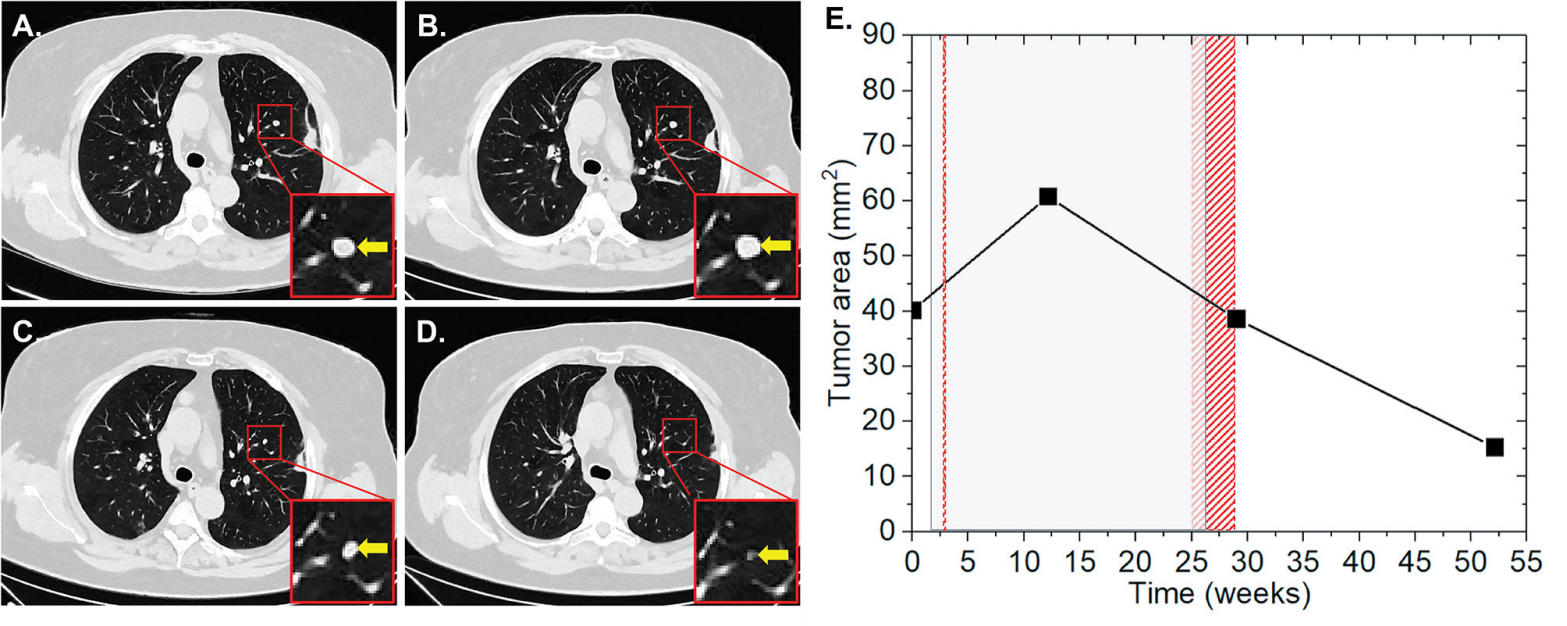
Patient 1 (Table 2B) presenting AbE in the lung. CT scans show the analyzed lesion (yellow arrows) before (A), 10 (B), 27 (C), and 50 weeks (D) after the induction of pembrolizumab. (E) The change in the lesion surface area with respect to the administration of IT (duration = 24 weeks, gray shaded area) and concurrent RT (red lines and area) of cerebral metastases with 1 × 20 Gy (1 week after 1st IT) and 25 × 2 Gy (23 weeks after 1st IT).
